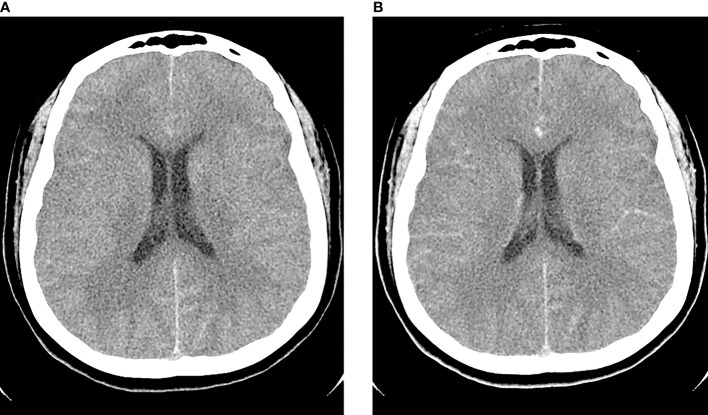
Axial pre-contrast (A) and post-contrast (B) computed tomography (CT) of the brain on day 3 post-infection showed generalized edema with post-contrast leptomeningeal enhancement.
